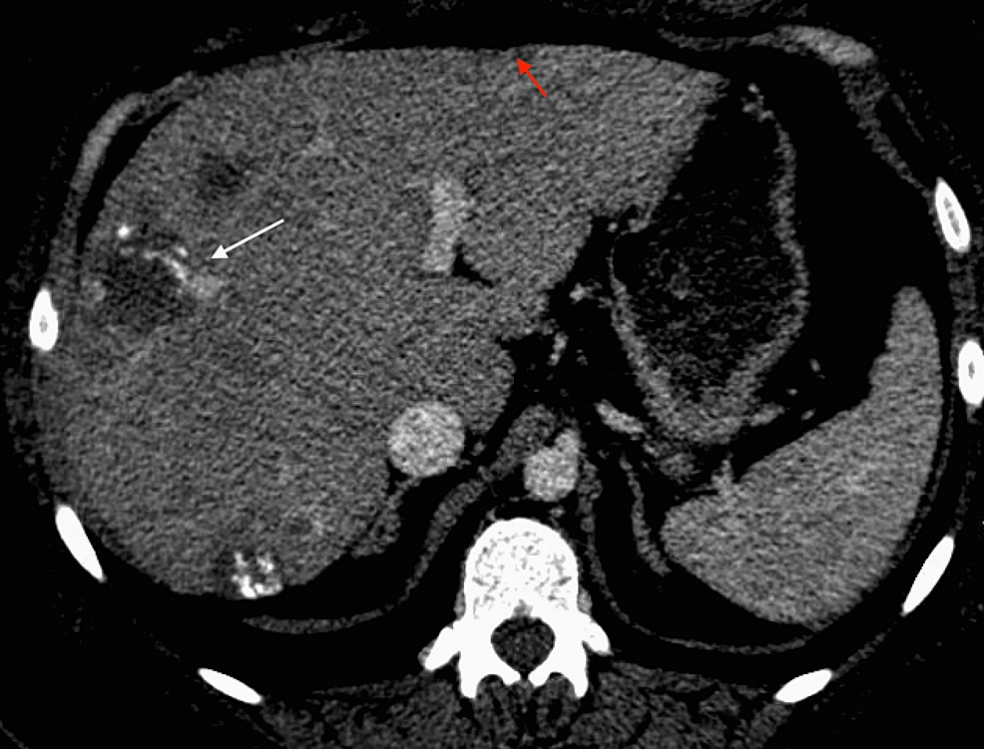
Axial view of a CT abdomen/pelvis showing capsular retraction (red arrow) as well as the “lollipop sign” (white arrow) CT: Computed Tomography
